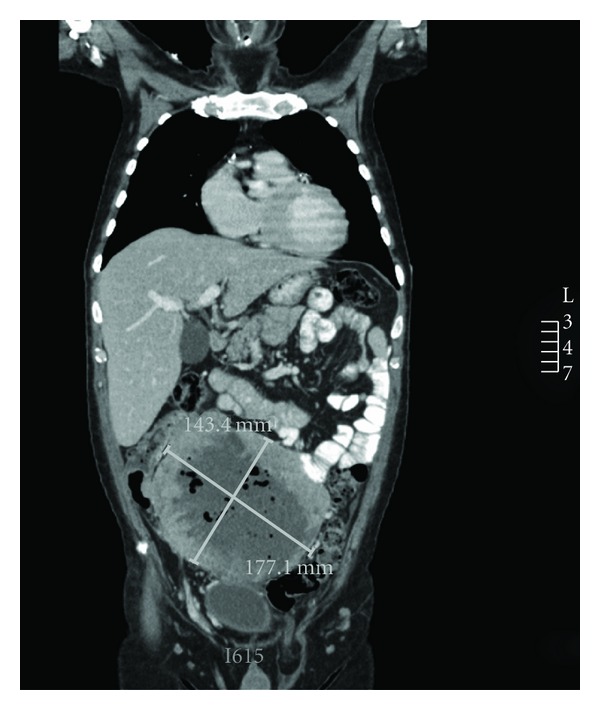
A representative cross-section of the mass on CT scan. The mass was secondarily infected following CT guided biopsy as indicated by the locules of gas within the tumour mass.
